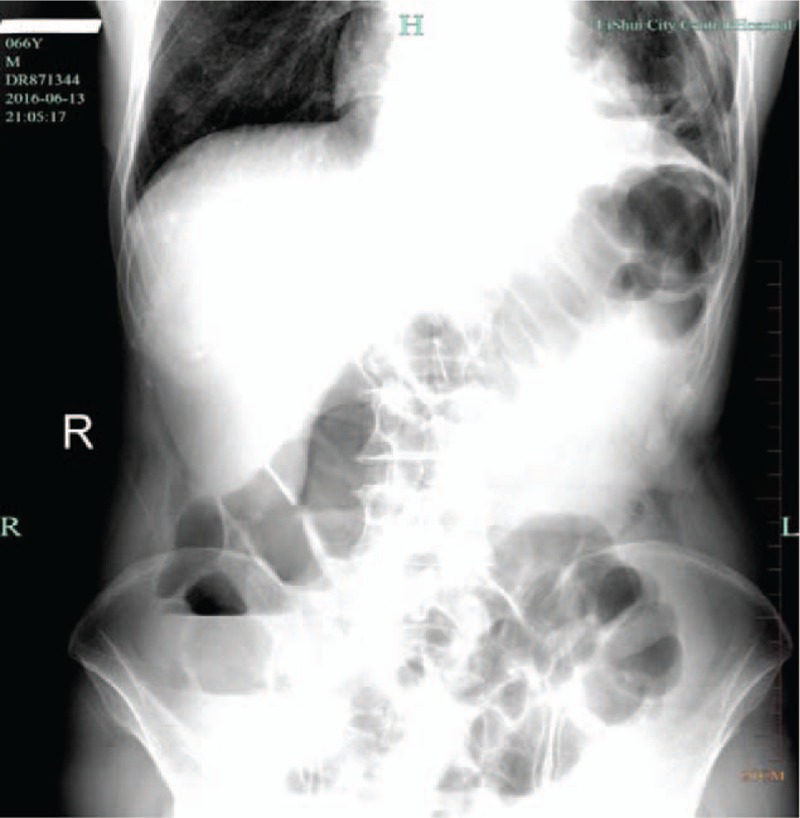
X-rays showed demonstrated several loops of dilated colon within the left upper quadrant.
